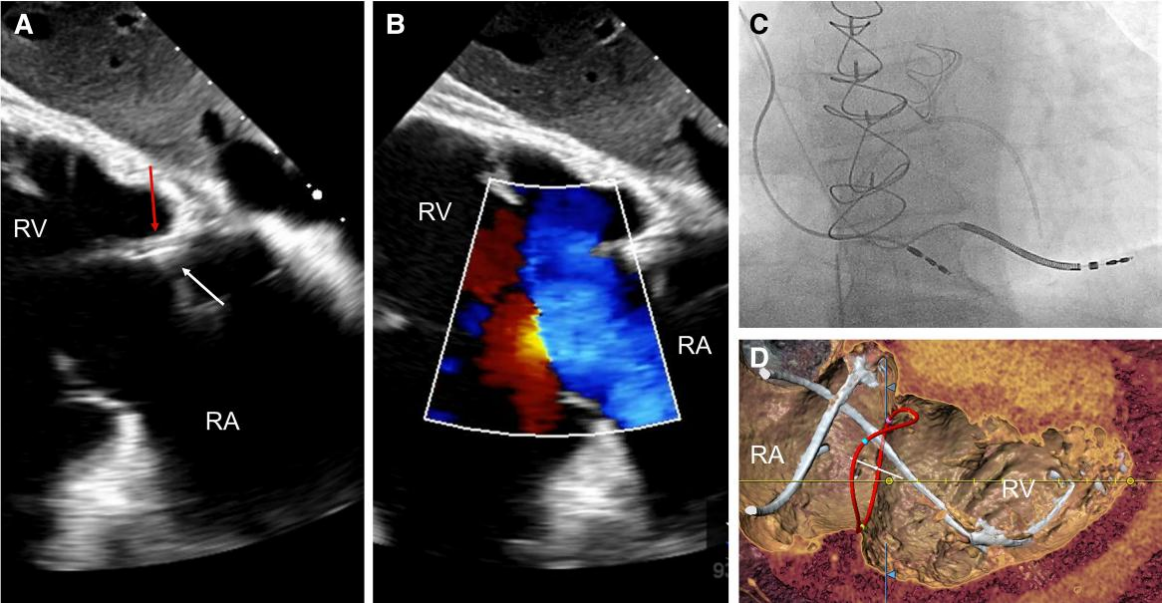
Example of a lead-related tricuspid regurgitation with ‘tricuspid kick’ and impingement of the posterior leaflet. Severe lead–leaflet interaction with impingement of the posterior leaflet resulting in severe cardiac implantable electronic device-related tricuspid regurgitation. (*A*) Impingement of the posterior leaflet; (*B*) severe tricuspid regurgitation with large coapation gap and secondary leaflet tethering; (*C*) excessive slack with visible tricuspid kick as a sign of potential interaction with the tricuspid annulus; and (*D*) cardiac computed tomography shows interaction with the tricuspid valve annulus (reconstructed line), as well as excessive slack in the right ventricle. RA, right atrium; RV, right ventricle.
